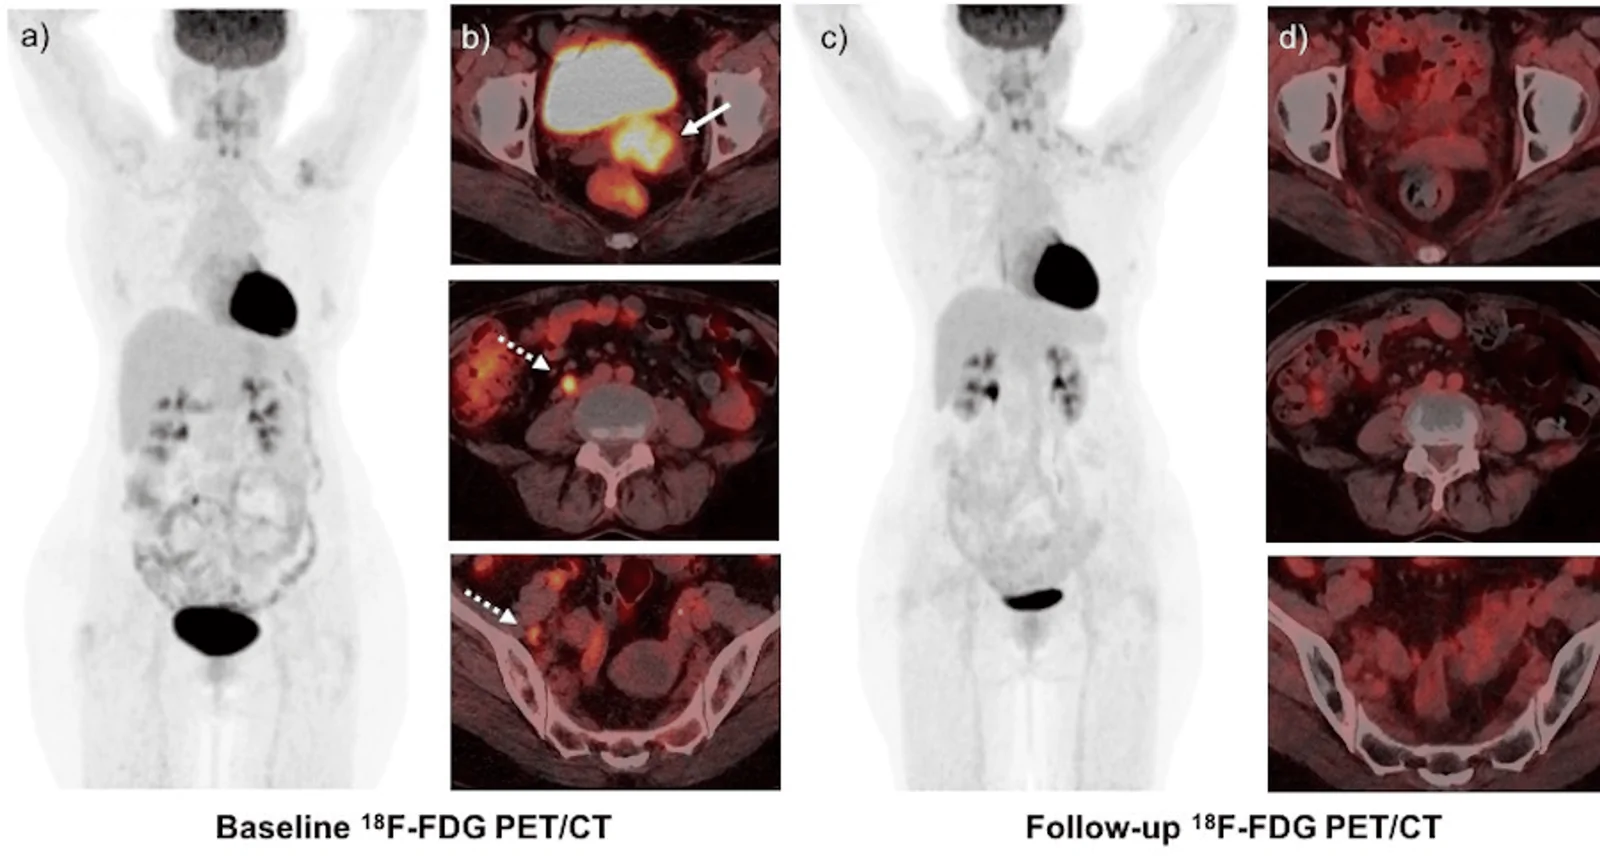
¹⁸F-FDG PET/CT in the treatment response assessment of cervical cancer Baseline (a) MIP and (b) fused ¹⁸F-FDG PET/CT images demonstrate an intensely ¹⁸F-FDG-avid primary cervical mass (solid arrow) with associated metastatic ¹⁸F-FDG-avid right common iliac and right external iliac lymph nodes (dotted arrow). Follow-up post-chemoradiation (c) MIP and (d) fused ¹⁸F-FDG PET/CT images show complete metabolic response, with the resolution of abnormal ¹⁸F-FDG uptake in both the primary cervical lesion and previously involved pelvic lymph nodes. ¹⁸F-FDG: ¹⁸F-fluorodeoxyglucose; PET: positron emission tomography; CT: computed tomography; MIP: maximum intensity projection
